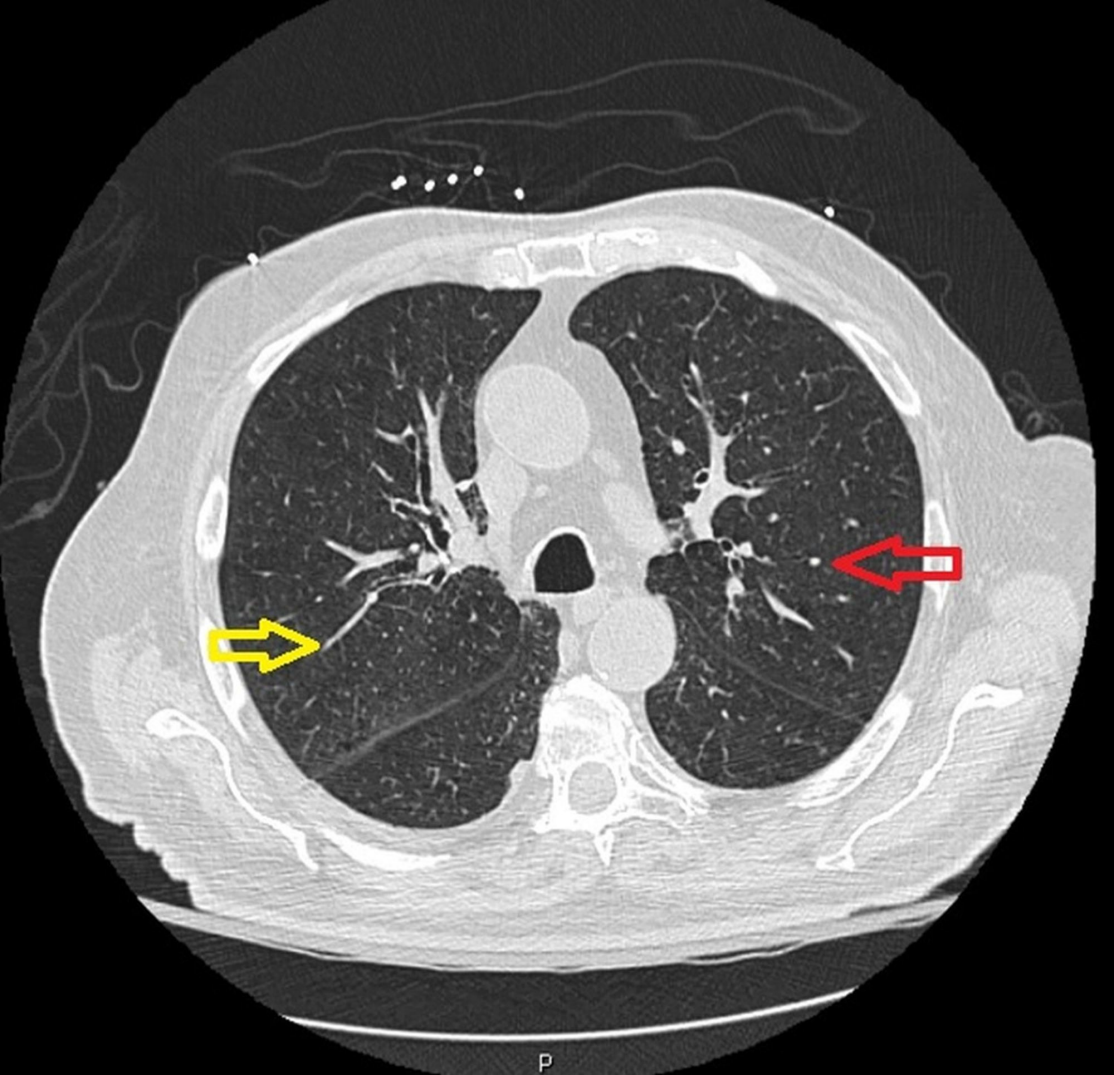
Non-contrast computed tomography scan of the chest showing bilateral pulmonary nodules (red arrow) and interstitial infiltrates (yellow arrow).
